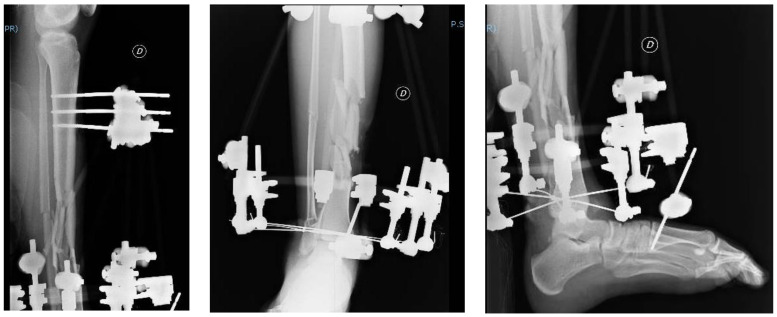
Post-operative radiographs; AP and LL projections of the right leg and ankle.
